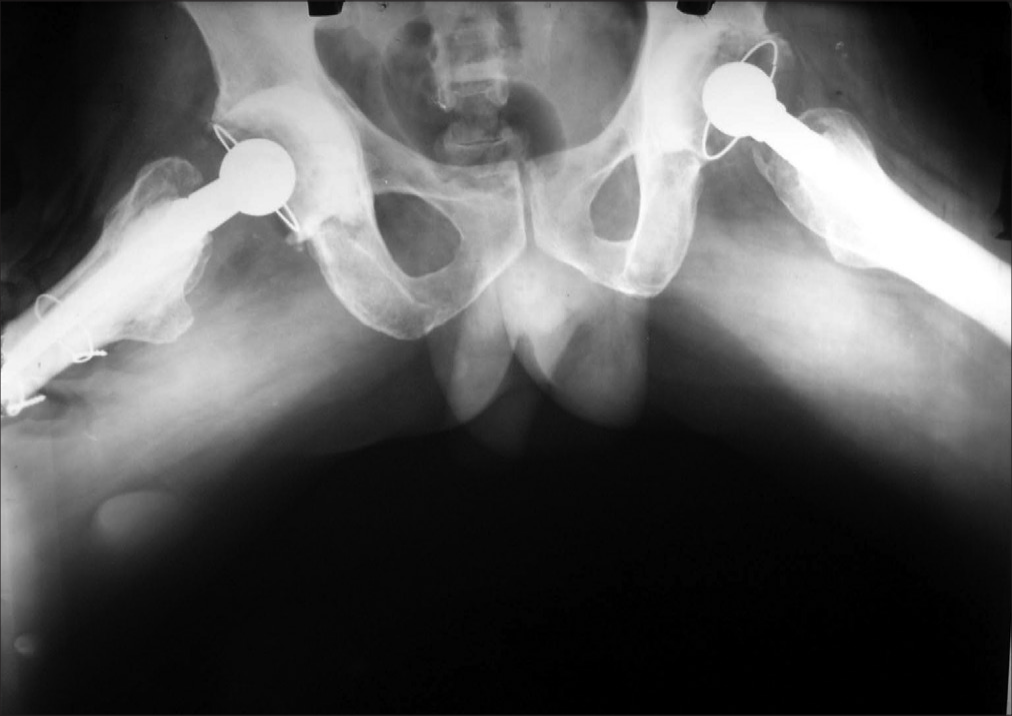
X-ray pelvis with both hips (frog lateral view) showing good range of movement at 11 years followup
